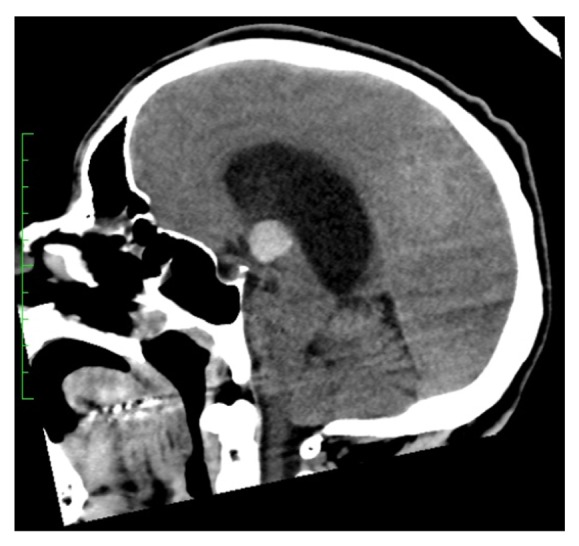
The marked lateral ventricle hydrocephalus secondary to a 16 x 15 x 15 mm colloid cyst within the third ventricle seen on CT scan at admission from the sagittal view.
